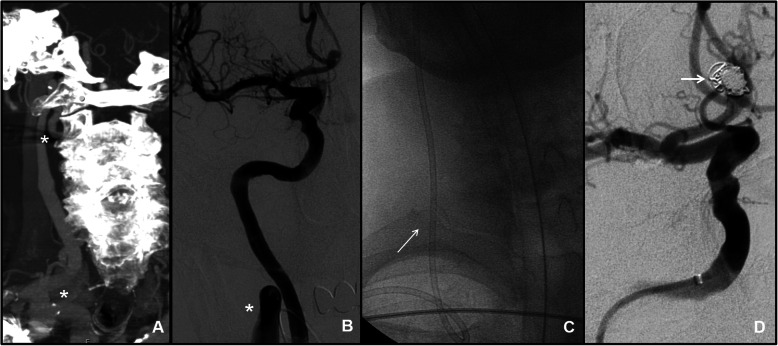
Sub-arachnoid hemorrhage with ACoA aneurysm in a 76-year-old man. Right trans-radial access was chosen because of a double kinking at CCA and ICA origin evaluated at CT-angiography and during DSA (asterisks in a and b). A 6 Fr guiding catheter was advanced for distal access into proximal ICA and simple coiling was obtained with compete aneurysmal occlusion (arrow in d)
